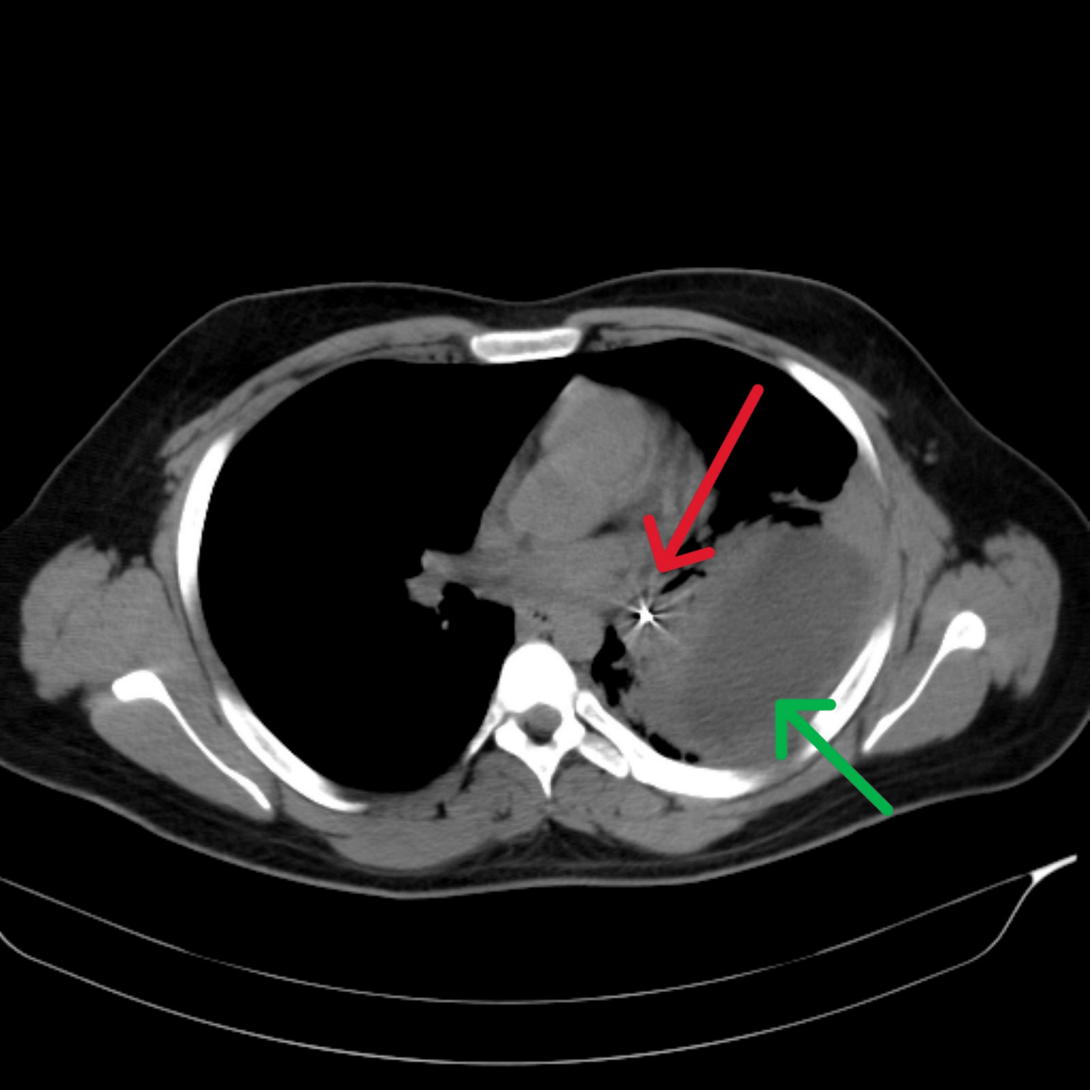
Axial section of a CT Chest. Case 4: A radio-opaque, long foreign body (pin) is seen in the left lower lobe bronchus (red arrow), causing collapse consolidation and resulting in a loculated effusion in the posterior aspect of the left thorax (green arrow).
